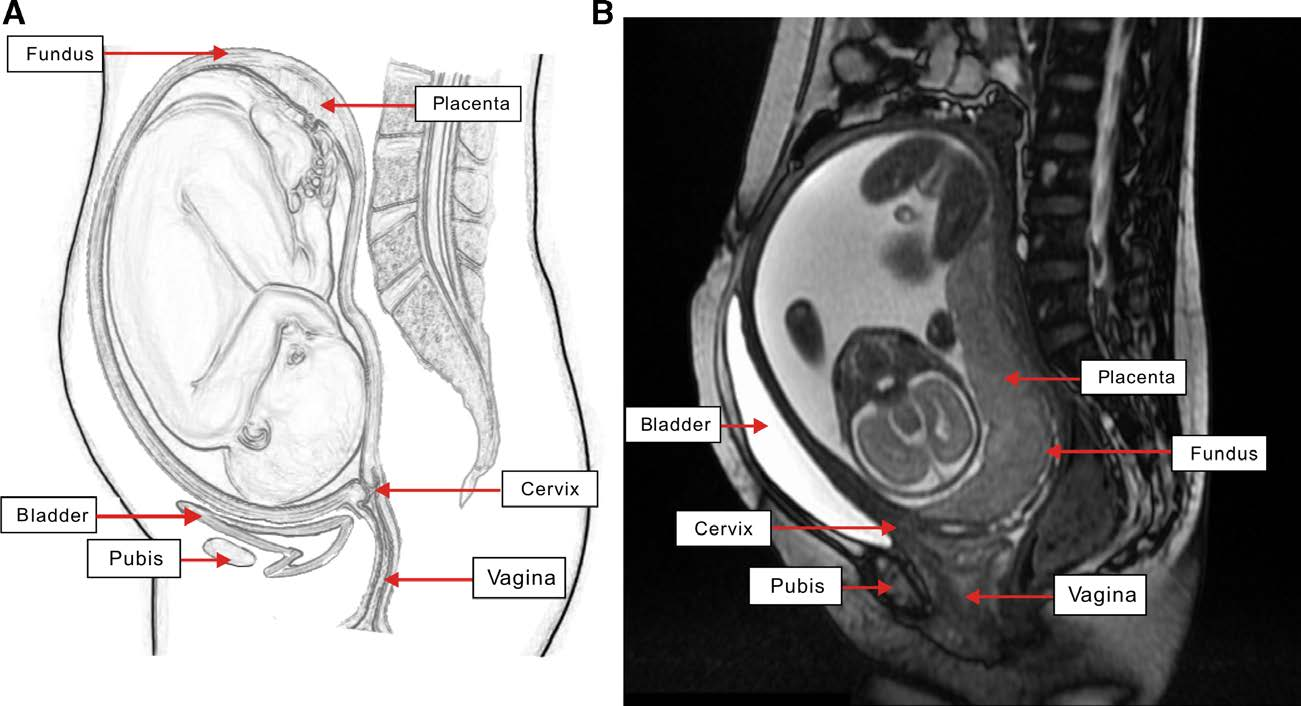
(A) Normal gravid uterus. (B) MRI of the first patient. The image shows the cervical orifice located in front of the anterior inferior wall of the uterus and the cervix and bladder was elongated. MRI = magnetic resonance imaging.
